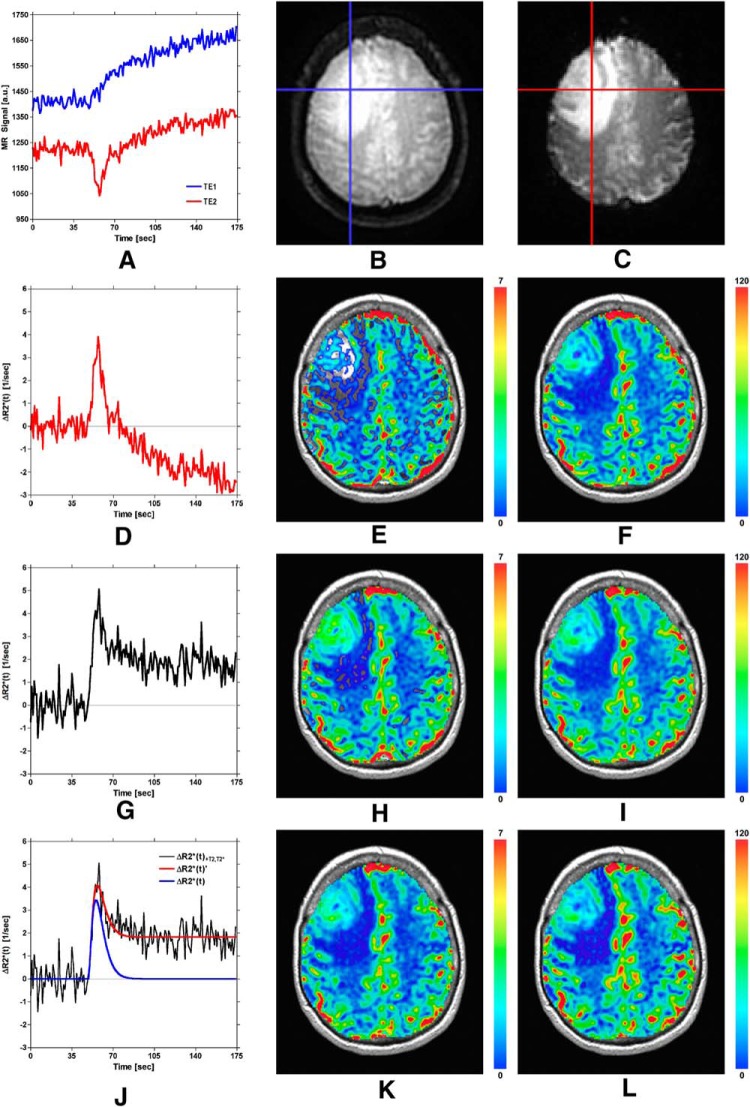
Demonstration of correction of DSC-MRI data using the proposed SPICE postprocessing method. See text for details.
